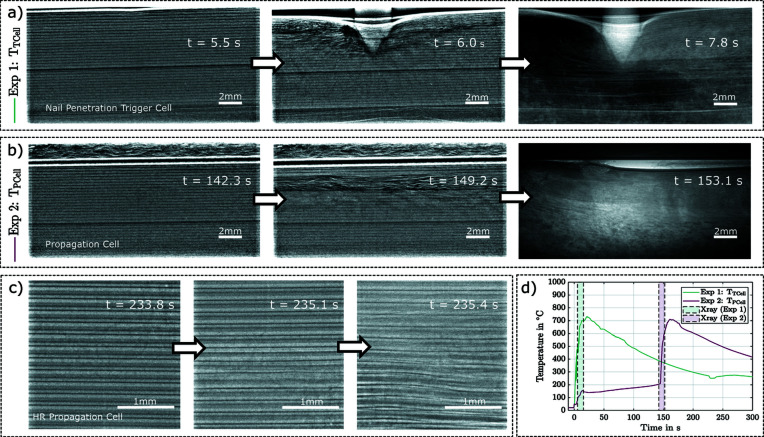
Example images from the high-speed X-ray recording. In Exp.1 (*a*) the cell was punctured directly with the nail, Exp.2 (*b*) and Exp.3 (*c*) show the cells directly under a punctured cell, which also entered a TR due to the temperature rise of the first TR. The setup of the images in (*c*) differs from that of (*a*) and (*b*) by a larger magnification factor (5×), resulting in a pixel size of approximately 4 µm. The images demonstrate the effects of a TR on the internal cell structure. To time the high-speed X-ray images in the abuse process, the images from Exp.1 and Exp.2 are plotted with the temperature data in (*d*). This facilitates the extraction of complex interrelationships across the entire process.
